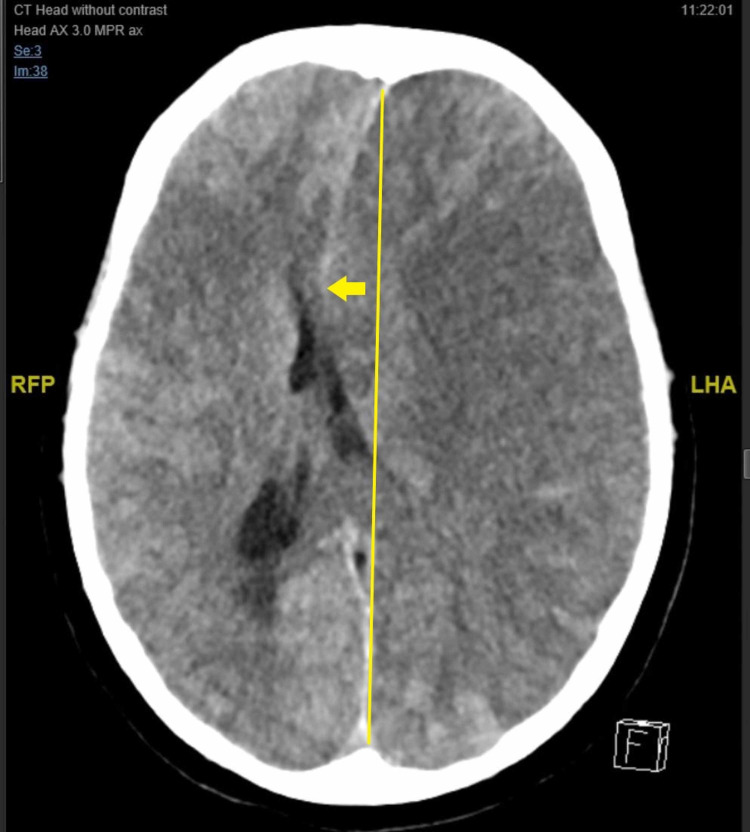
CT brain without contrast - right midline shift
